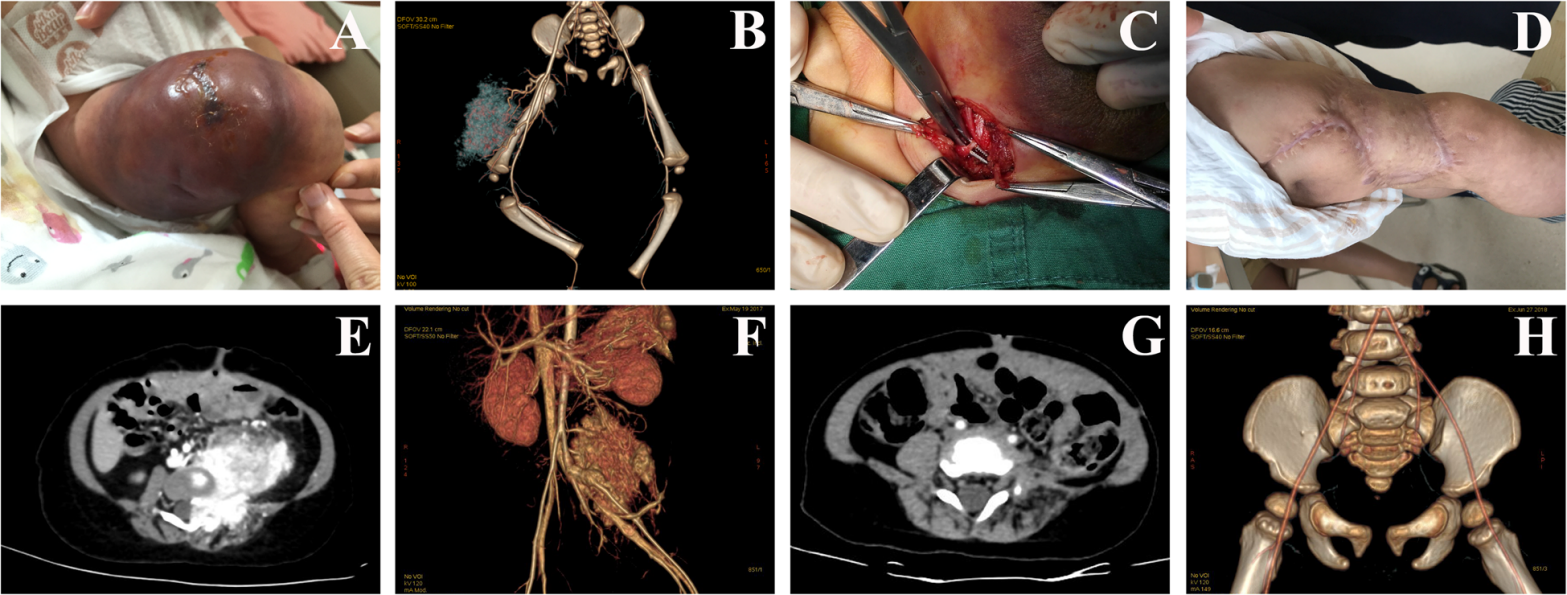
a: 1 month old baby’s right thigh with a huge KHE and KMP, the tumor occupied the outer side of the thigh. b: Three-dimensional reconstruction of CTA showed the tumor was feeding by the right deep femoral artery and its branches. c: In the operation, a part of the tumor feeding artery was ligated to reduce intraoperative bleedingat first. d: 1 year after tumor resection and skin grafting, the patient’s wound healed well and no recurrence was seen. e: Pelvic cavity CT scan of a 9-month-old baby showed a soft tissue mass in the left pelvic cavity. The boundary between the mass and the left psoas muscle and the iliopsoas muscle was unclear. The spinal canal was invaded, and the left side of L3–5 vertebrae structure was destroyed. Contrast-enhanced CT scan showed significant enhancement within the tumor. f: Three-dimensional reconstruction of CTA showed the tumor was closely adhered to the abdominal aorta and the left internal iliac artery. The tumor was compressed and partially wrapped around the left external iliac artery. g: 1 year after surgery, pelvic CT showed L3–5 vertebral structurewas partially repaired, and no recurrence was seen. h: 1 year after surgery, CTA showed no tumor was wrapped in the left external iliac artery, and no filling defect was seen in the lumen
